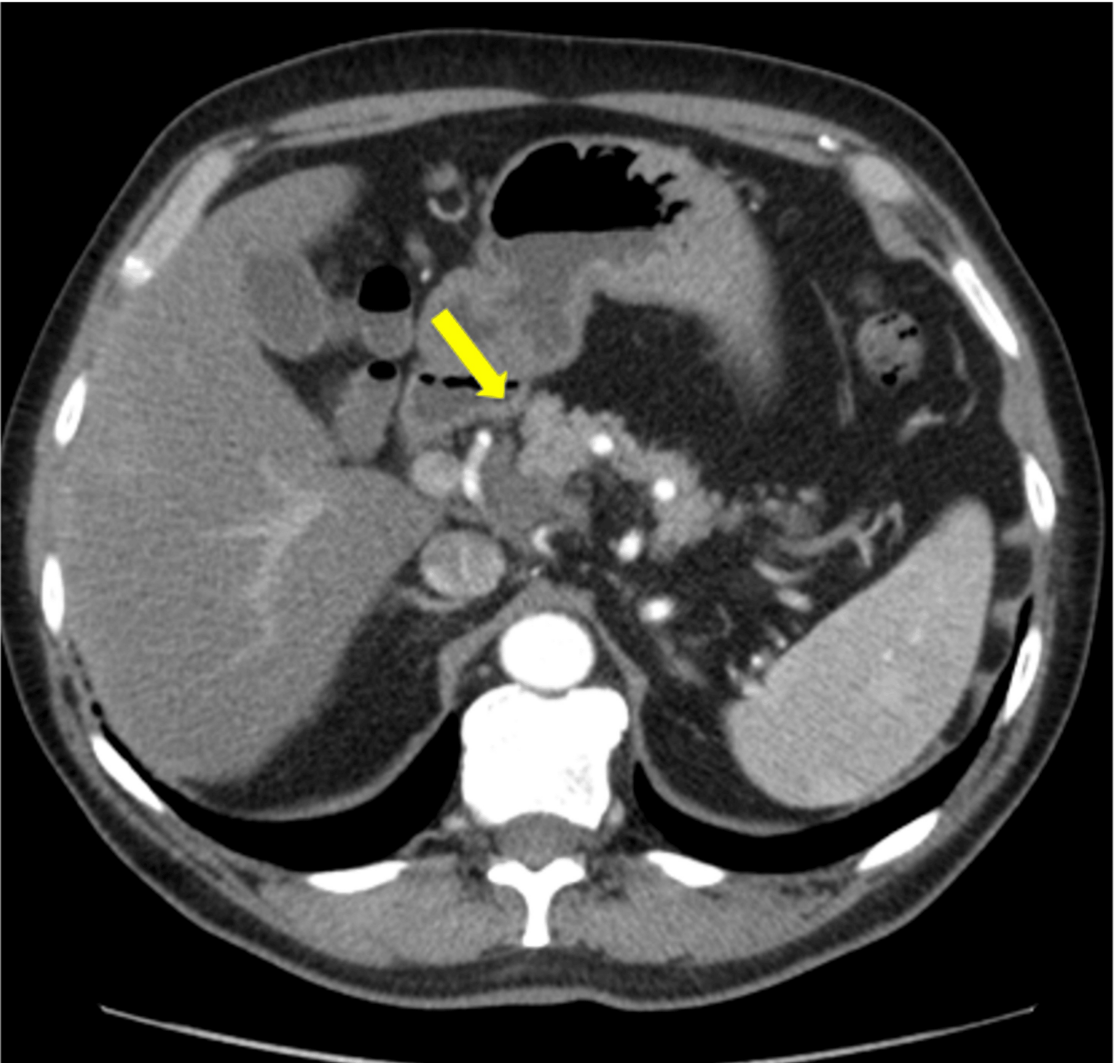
Axial contrast-enhanced CT following chemotherapy showing marked reduction of the soft-tissue density around the common hepatic artery (yellow arrow), consistent with excellent response of the primary tumour.
